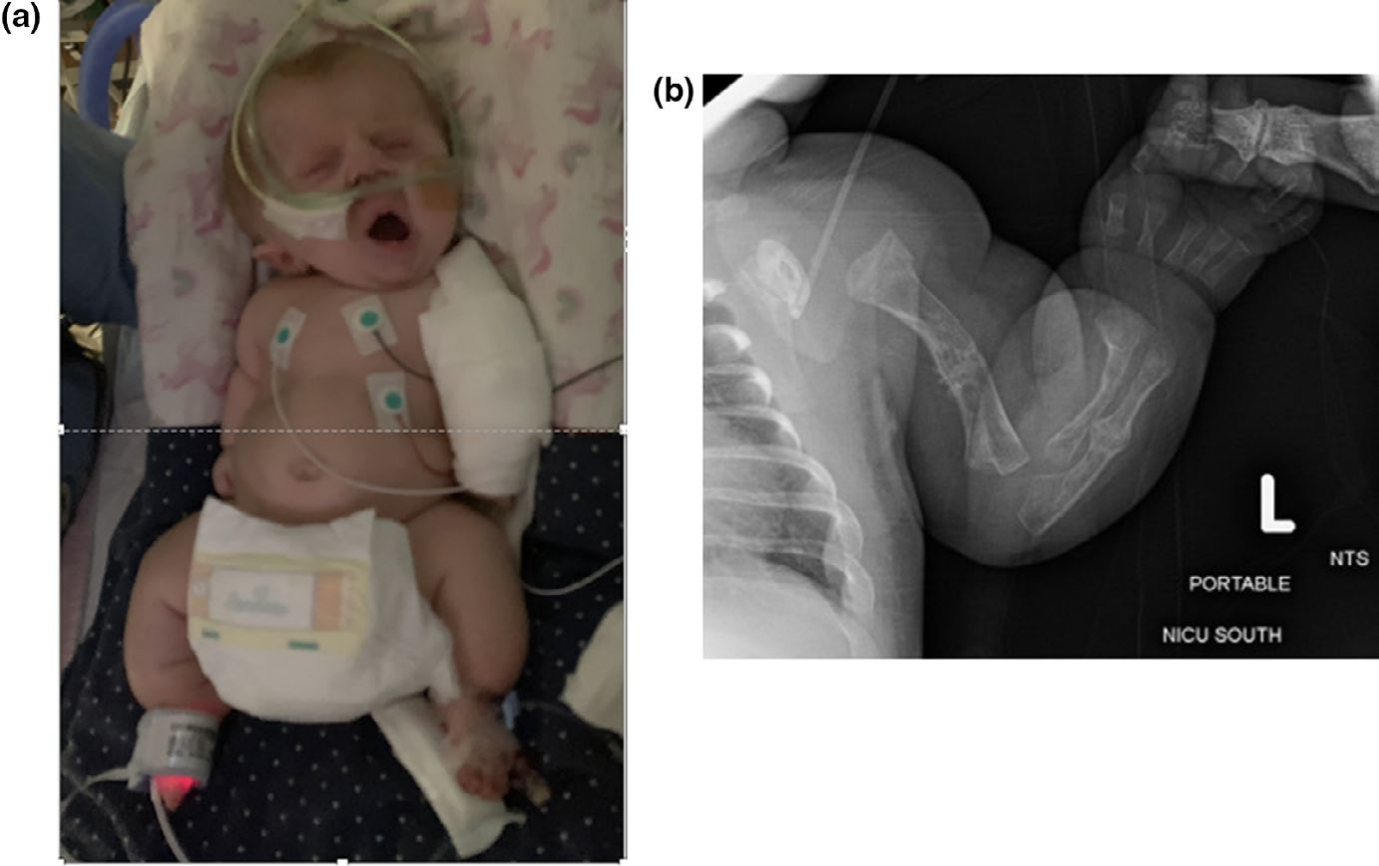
a, 4‐month‐old girl with a cotton wrap on the left upper extremity for a presumed left upper extremity fracture. b, Left upper extremity radiograph from 8 days of age; radiographs of the left upper extremity were not pursued with fracture at 4 months of age; wrap was removed approximately 10 days following the fracture with resumption of baseline comfort level and movement and use of the left upper extremity. Photograph used with parental consent
